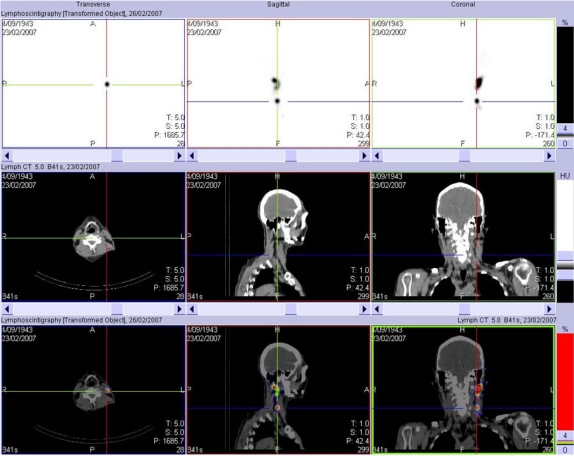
The high tumour to background ratios obtained with agents such as Tc-99m antimony colloid, used for lymphoscintigraphy, limit the anatomical localisation of abnormalities on SPECT alone. Fusion of SPECT and CT data redresses this limitation. The ability to demonstrate to the surgeon the precise location of the sentinel node means that the correct node is more likely to be sampled and that operative time and morbidity may be reduced by better surgical planning.
